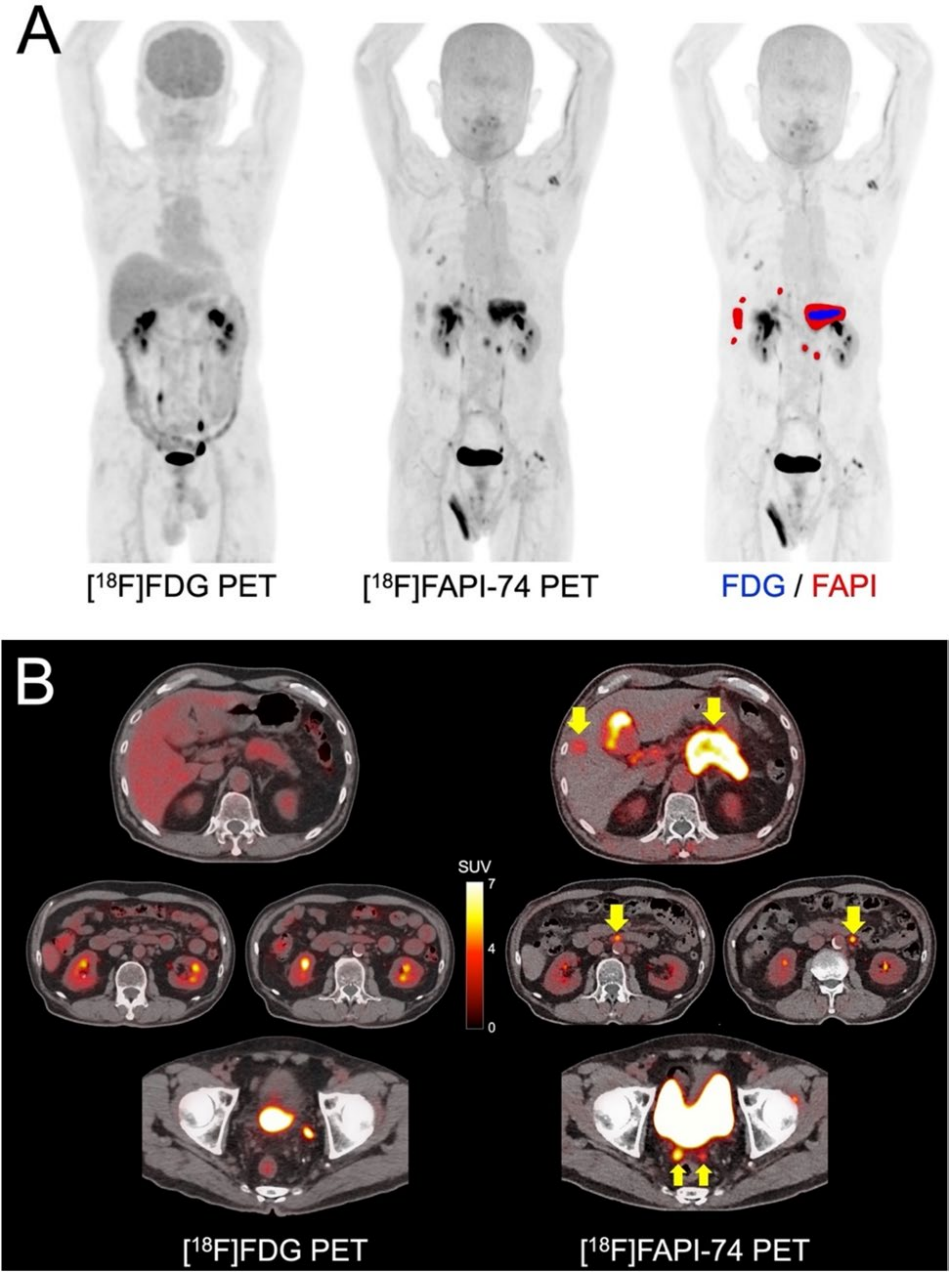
68-year-old male patient with multiple lymph node and peritoneal metastases of pancreatic cancer. A Comparison of the maximum intensity projection (MIP) images: the image on the right shows fusion with positive lesions on [^18^F]FAPI-74 PET (red-colored area) and [^18^F]FDG PET (blue-colored area), B PET/CT fusion images on [^18^F]FAPI-74 PET and [^18^F]FDG PET (arrows indicated metastatic lesions). [^18^F]FAPI-74 PET detected more metastatic lesions compared with [^18^F]FDG PET (SUVmax of the primary lesion is 9.4 and 3.2, respectively). (Cited from reference no. 40 in accordance with the open access policy.)
